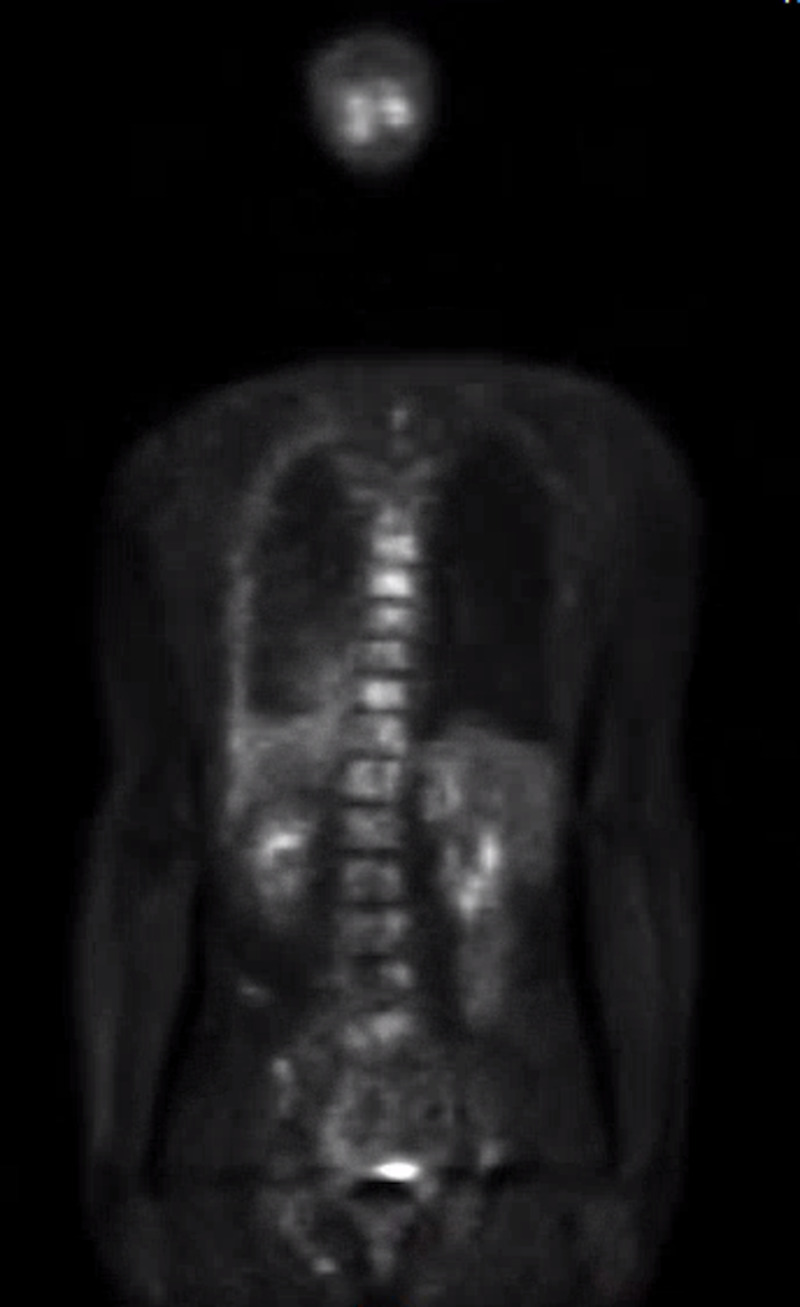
Positron emission tomography/computed tomography (PET/CT) did not reveal primary renal cell carcinoma
